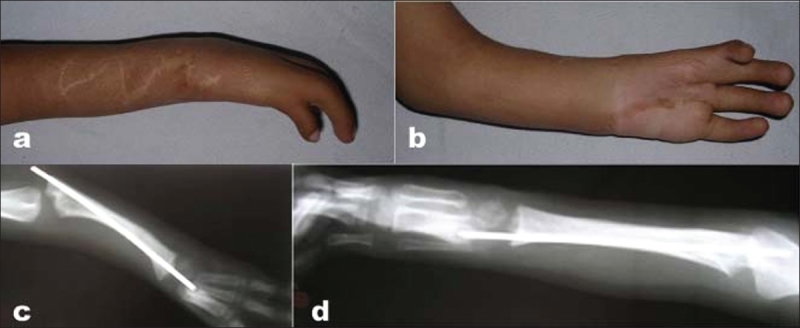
Case no 9 (a,b) clinical photograph of the same patient shown as figure 1 showing correction at 1 year follow up. (c,d) X-ray of correction of same patient at 1 year
